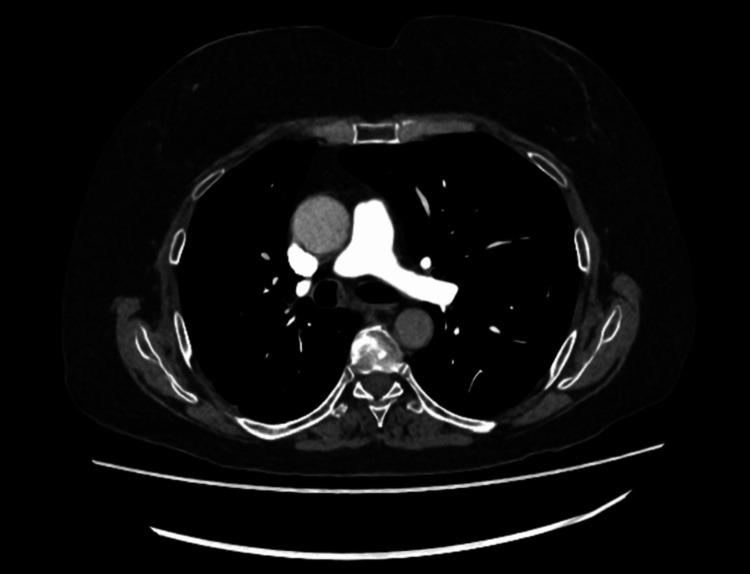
CT pulmonary angiogram demonstrating no pulmonary embolism. No new nodules or effusions were observed. This imaging confirmed disease stability and excluded thromboembolic pathology as the cause of the patient’s respiratory symptoms.
